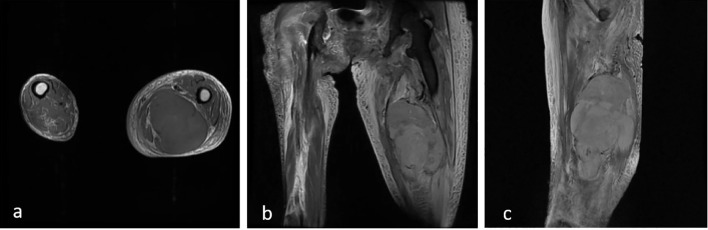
Sequential MRI in axial (a), coronal (b) and sagittal (c) views showed a huge mass in the posteromedial aspect of the thigh.
